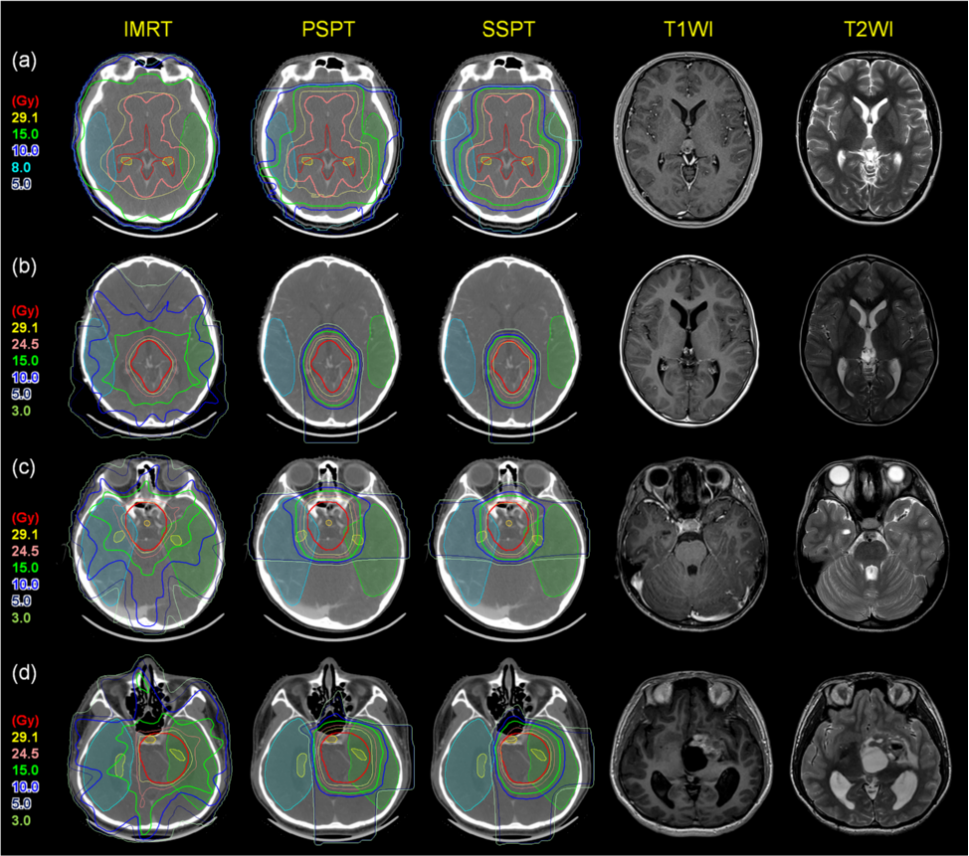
Dose distributions of WV irradiation and PG, SS and BG tumor beds with their pre-chemotherapy MRI images. Thick pink line is WV PTV, and thick red lines are tumor beds PTV. Prescribed dose are 30.6 Gy at 95 % volume of PTV. (a) WV (b) PG (c) SS (d) BG
